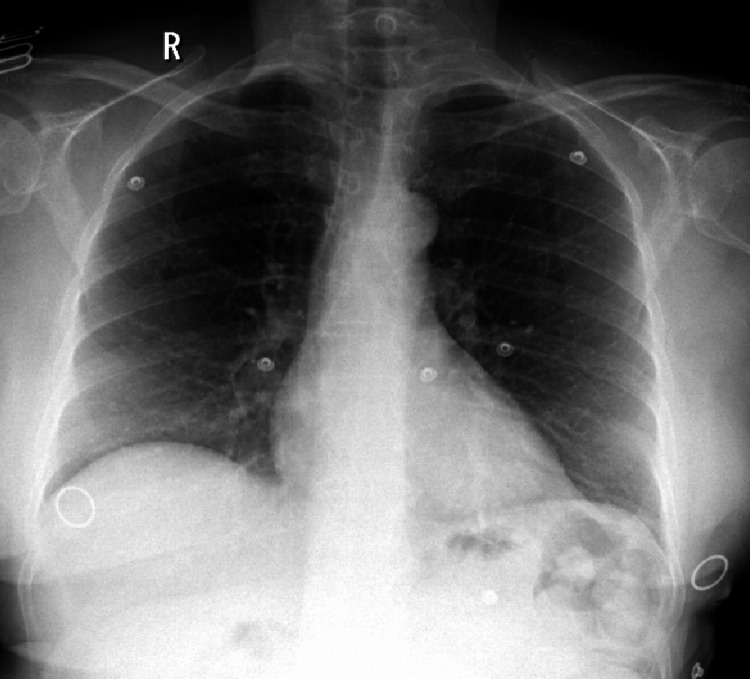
Chest X-ray on re-admission The repeat chest X-ray was noted to be normal.
